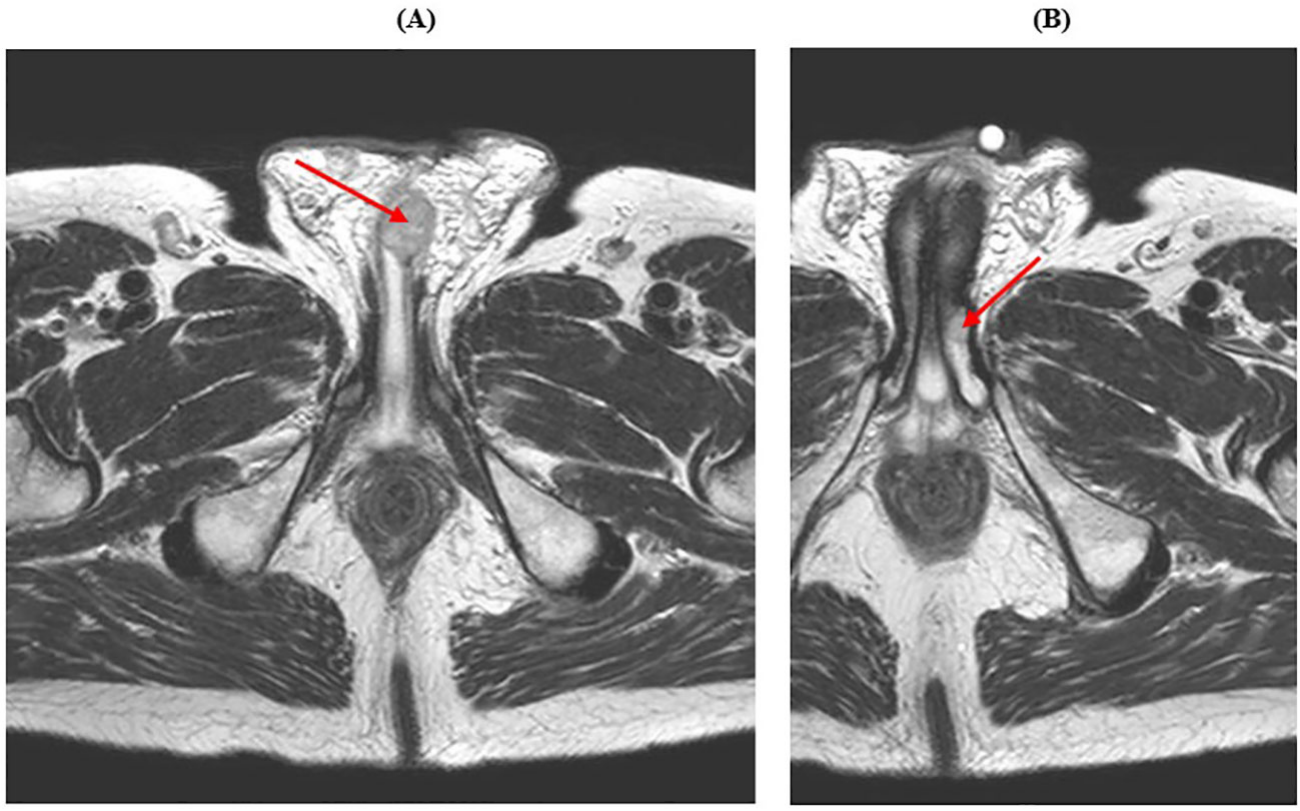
Pelvic MRI showing male genital system metastases. (A) The red arrow indicates the lesion in the corpus spongiosum. (B) The red arrow indicates the lesion in the corpus cavernosum.
